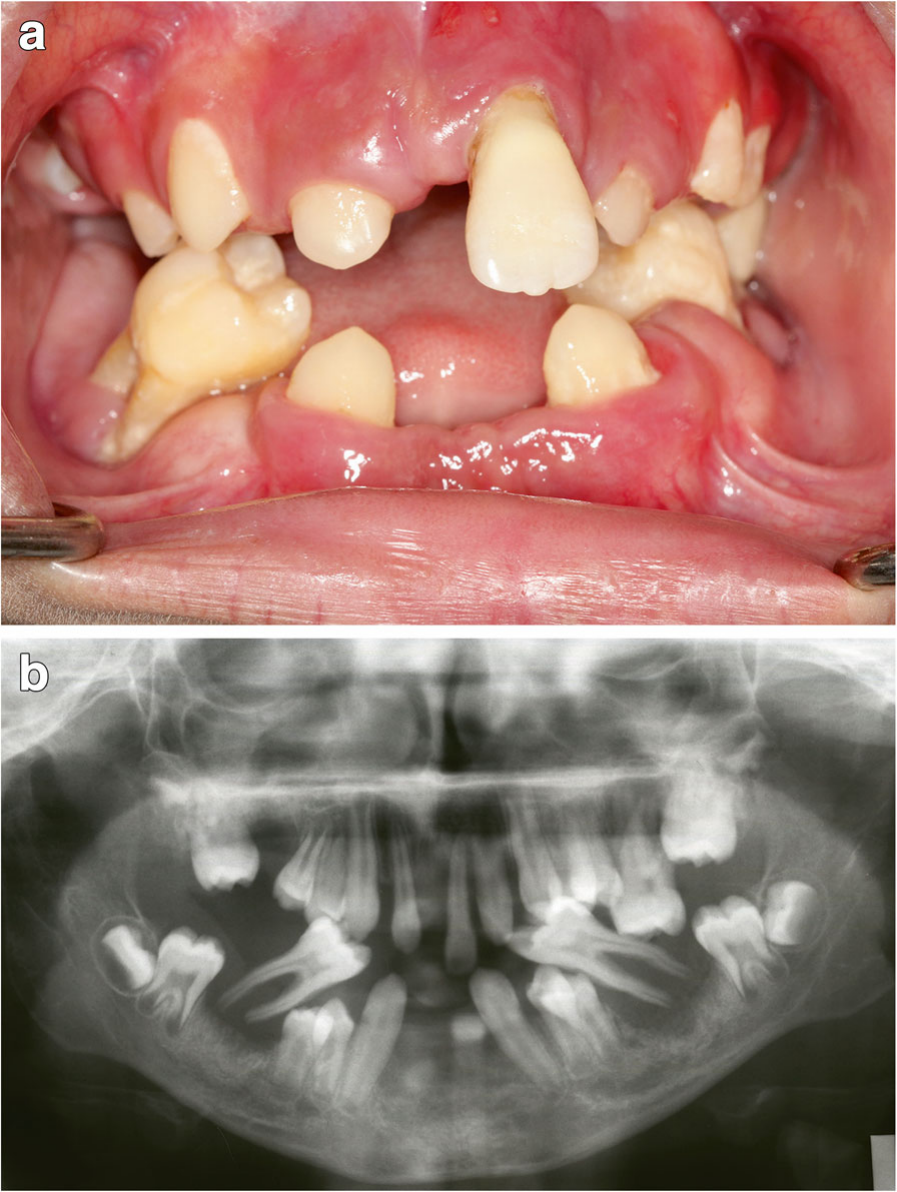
Case 4. a Severe gingivitis with periodontal destruction and migration of permanent molars. b Panoramic radiograph showing severe periodontal destruction, note the migration and floating of the lower first molars
